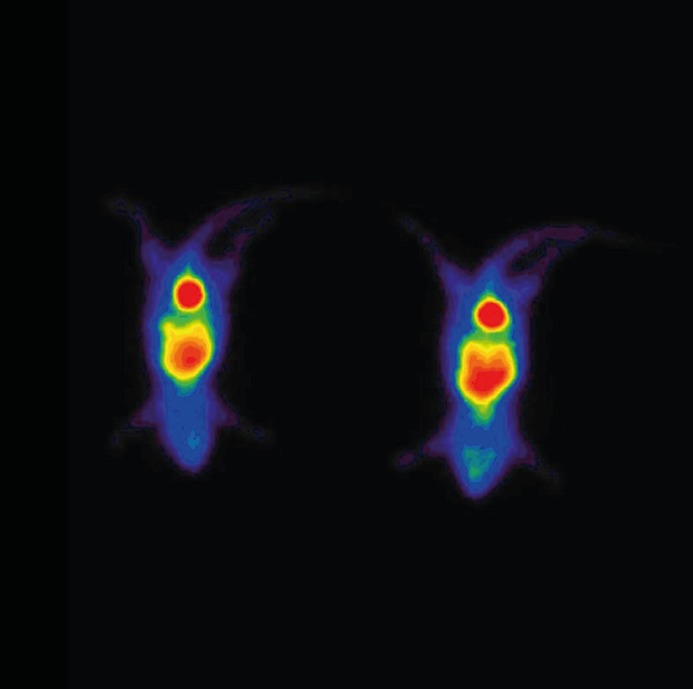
Planar SPECT imaging of the [^99m^TC]-ECB-DG complex after 2 hours administration
